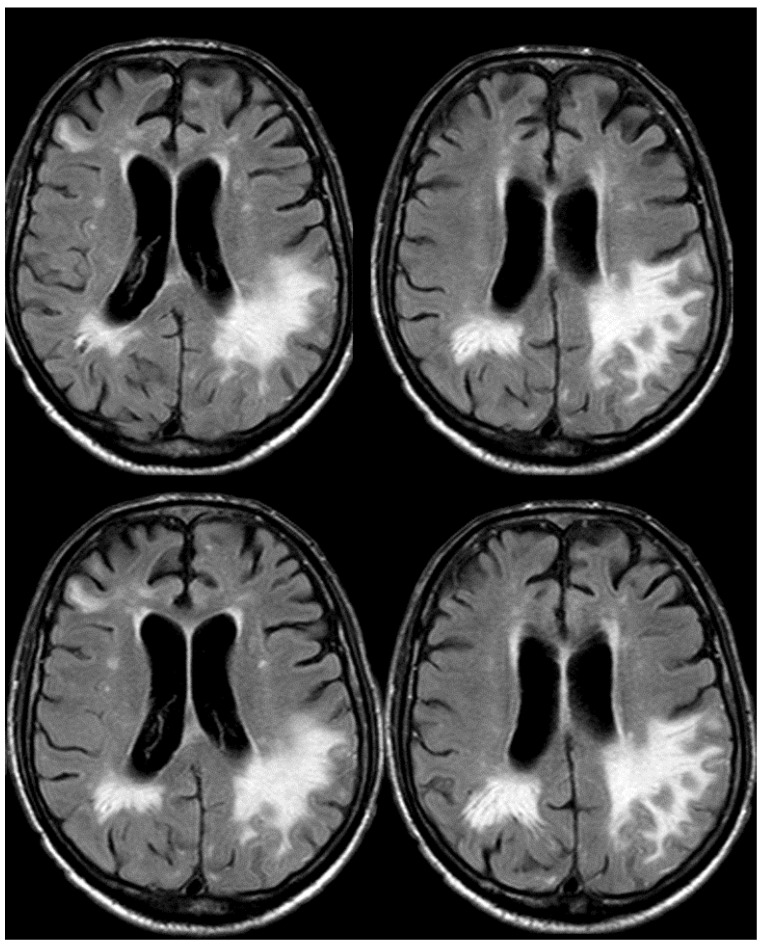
Brain MRI (axial FLAIR) before and after contrast administration (upper and lower row, respectively), showing a tumefactive white matter hyperintense signal change in the left posterior frontal and parietal lobe, sparing the U fibers and with DWI/ADC features of vasogenic edema (not shown) without contrast enhancement.
